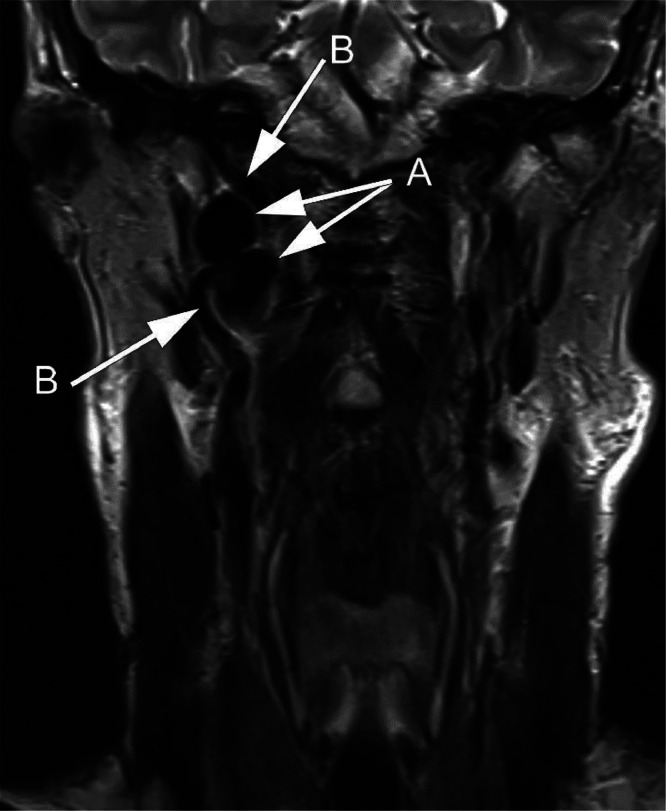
Coronal T2 W MR image through the neck demonstrates a bilobar mass, appearing of signal void (A), in the upper right neck near the skull base, the internal carotid artery can be seen entering and leaving the lesion (B).
